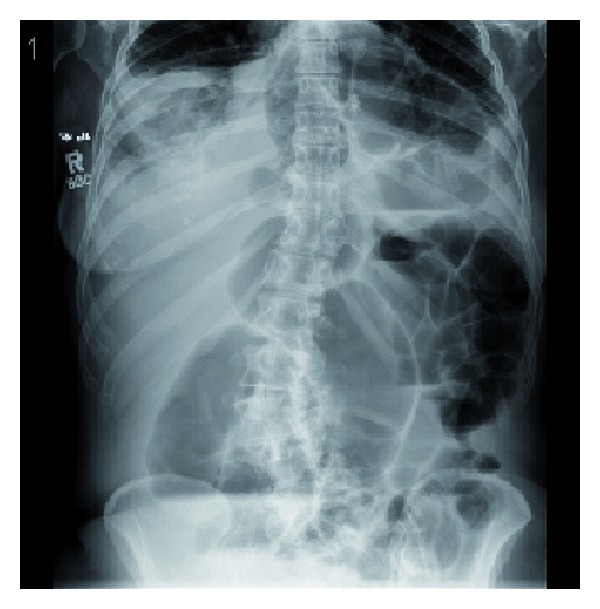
Abdominal radiograph reveals air distended loops of descending and transverse colon. Additionally, a loop of bowel may be seen extending into the pericardial space.
